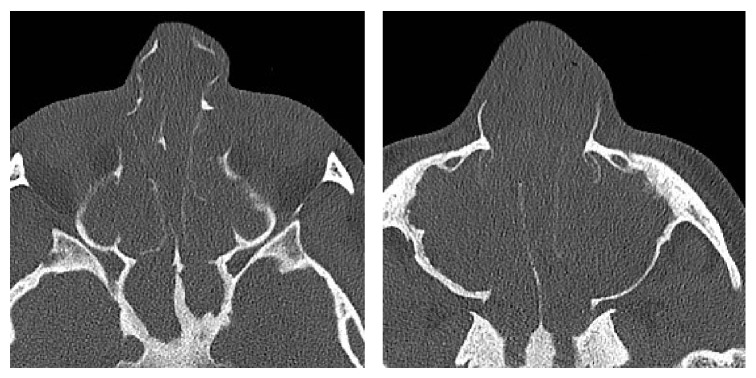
CT scan showing severe atrophy and deformity of the nasal pyramid.
